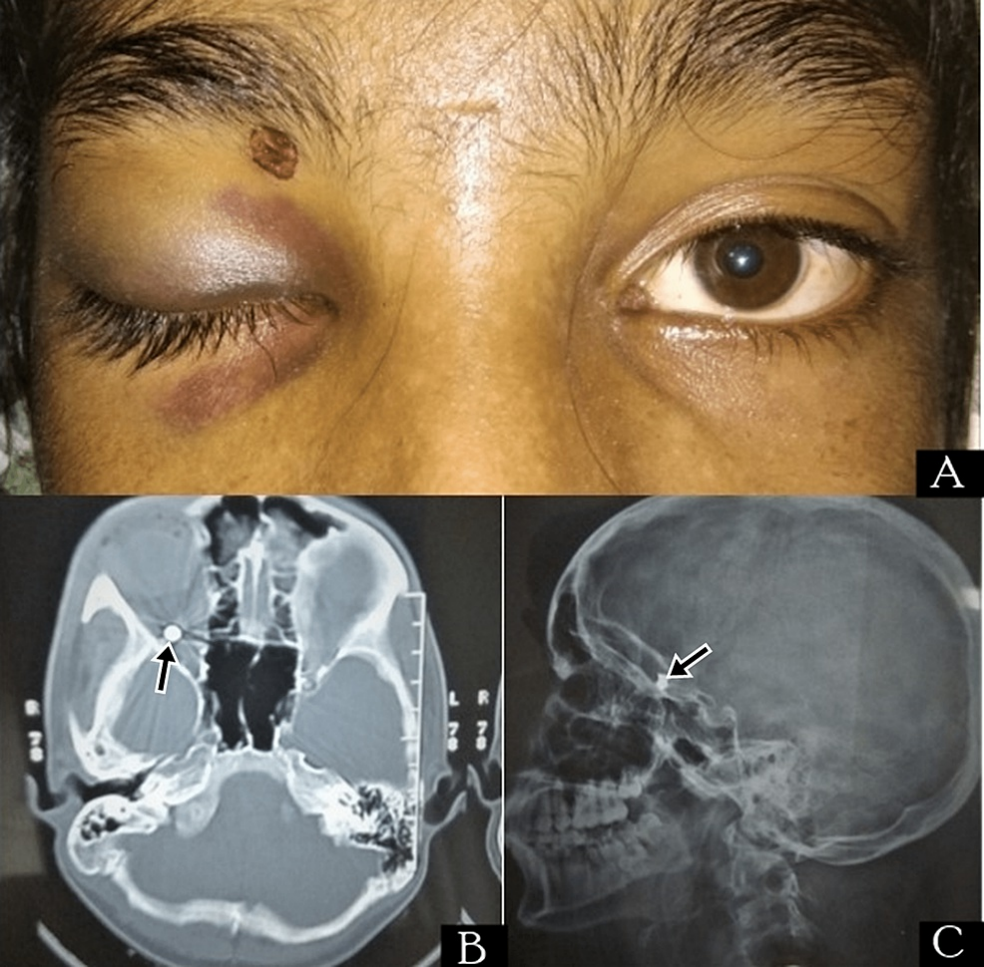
Case 2 (A) Right eye ecchymosis, ptosis and small right upper lid wound; (B) X-ray showing foreign body (FB) in intraconal space; (C) non-contrast computed tomography (NCCT) showing FB in the orbital apex.
